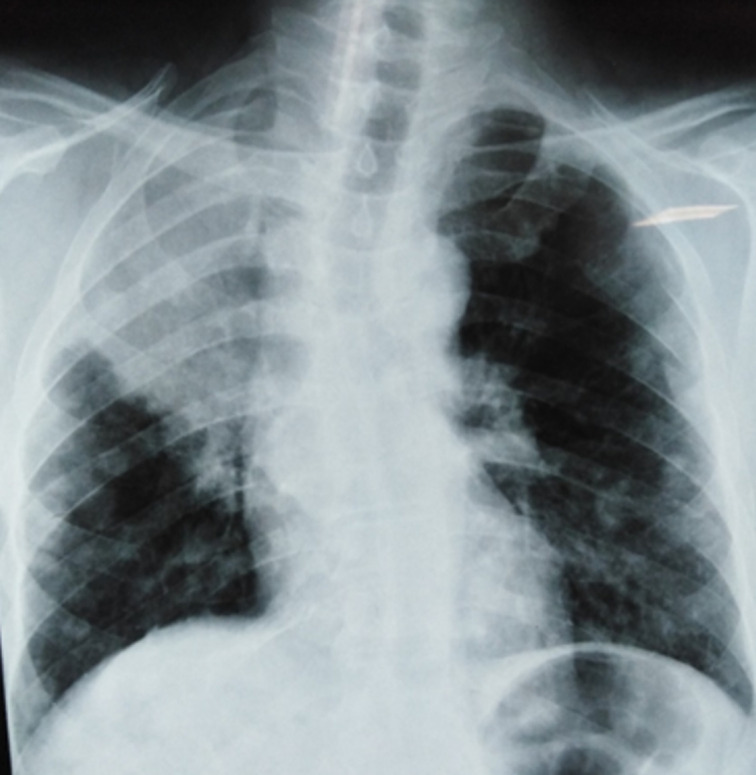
radiographie thoracique de face opacité pulmonaire droite avec des nodules bilatéraux
